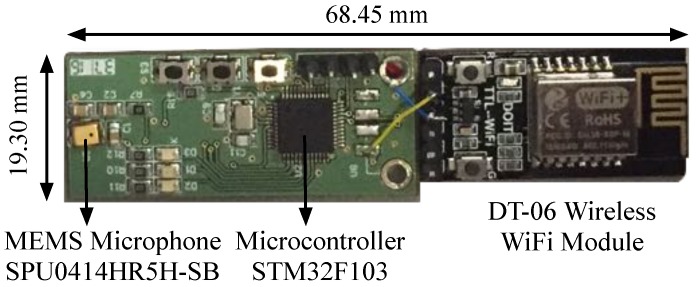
Ultrasonic receiver module.
